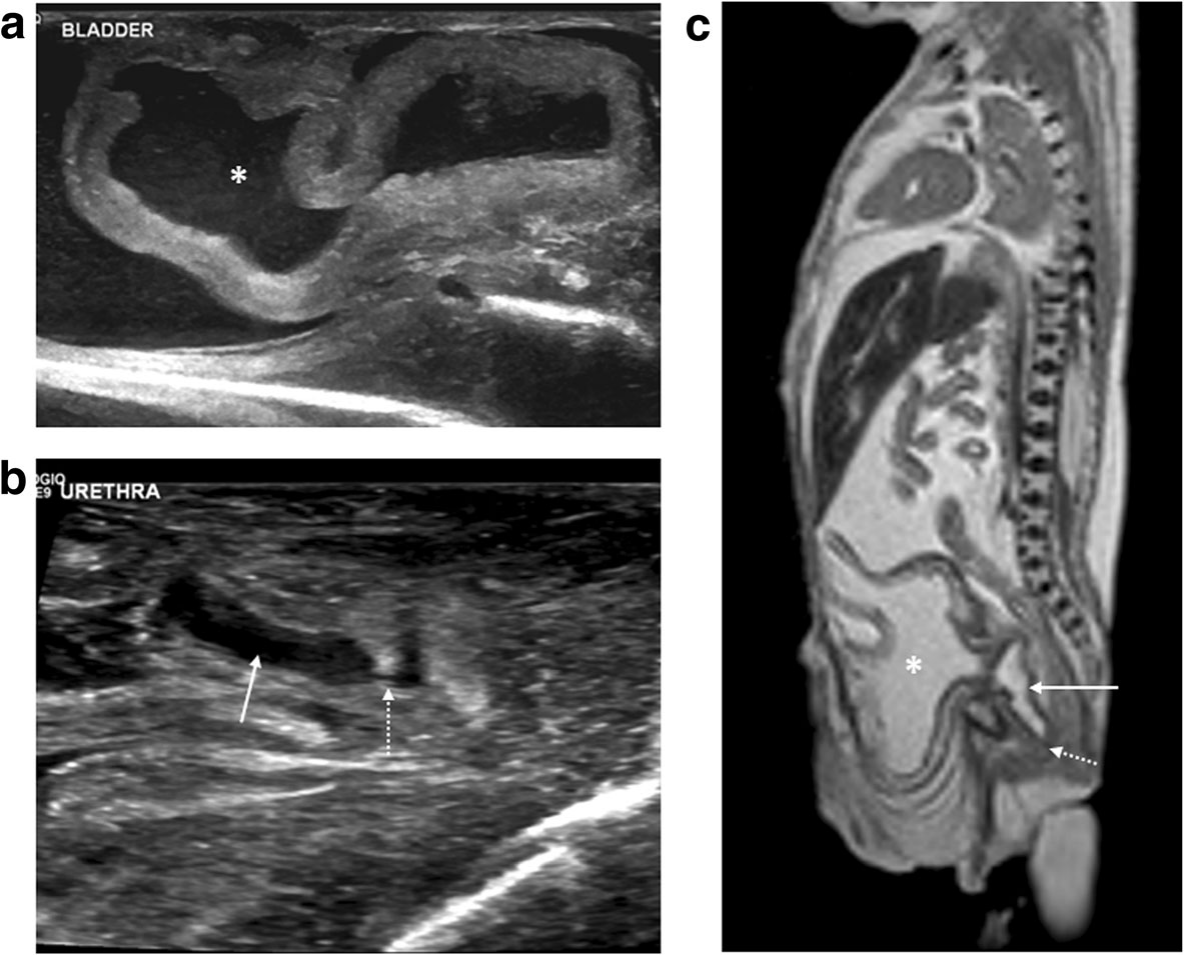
Bladder outlet obstruction due to posterior urethral valves in a foetus at 21 weeks gestation. The post-mortem imaging was obtained three days after termination of pregnancy. Sagittal post-mortem ultrasound images of the bladder (a) and posterior urethra (b) demonstrate a partially distended urinary bladder (asterisk) with thickened bladder wall, a dilated posterior urethra (solid arrow) and an abrupt urethral narrowing (dotted arrow) at site of obstruction. The corresponding sagittal post-mortem T2 weighted MRI study (c) also demonstrates the large urinary bladder (asterisk) with dilated posterior urethra (solid arrow), although the site of obstruction (dotted arrow) is less well depicted than on the ultrasound
